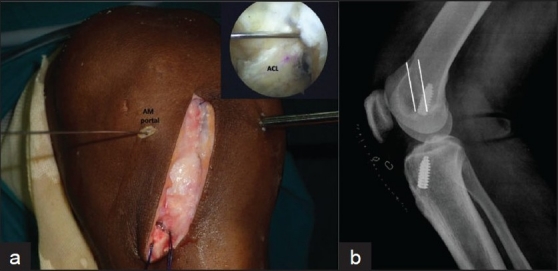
(a) Guide wire through AM portal showing divergence in the arthroscopic image (inset) in the left knee. (b) Lateral X-ray of knee showing interference screw divergent to femoral tunnel (parallel white lines depict outline of femoral tunnel)
